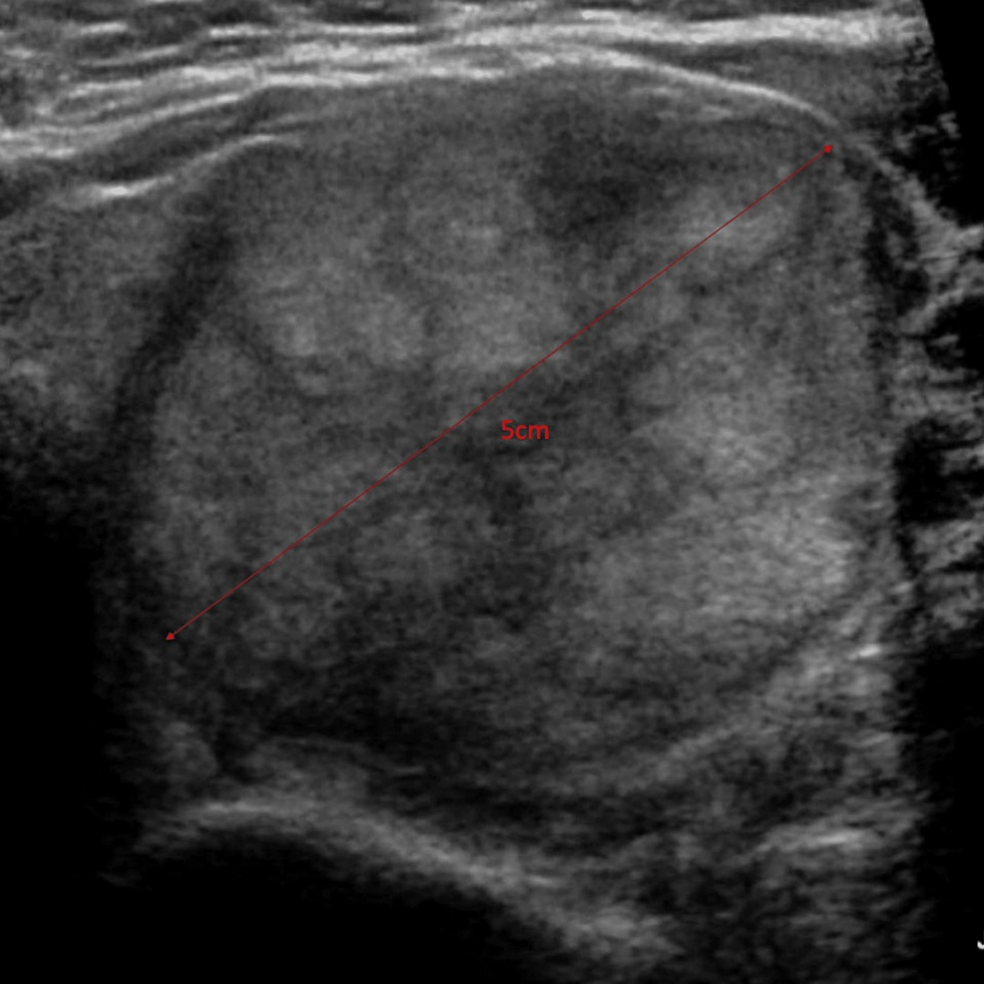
5.0 cm nodule on ultrasound
